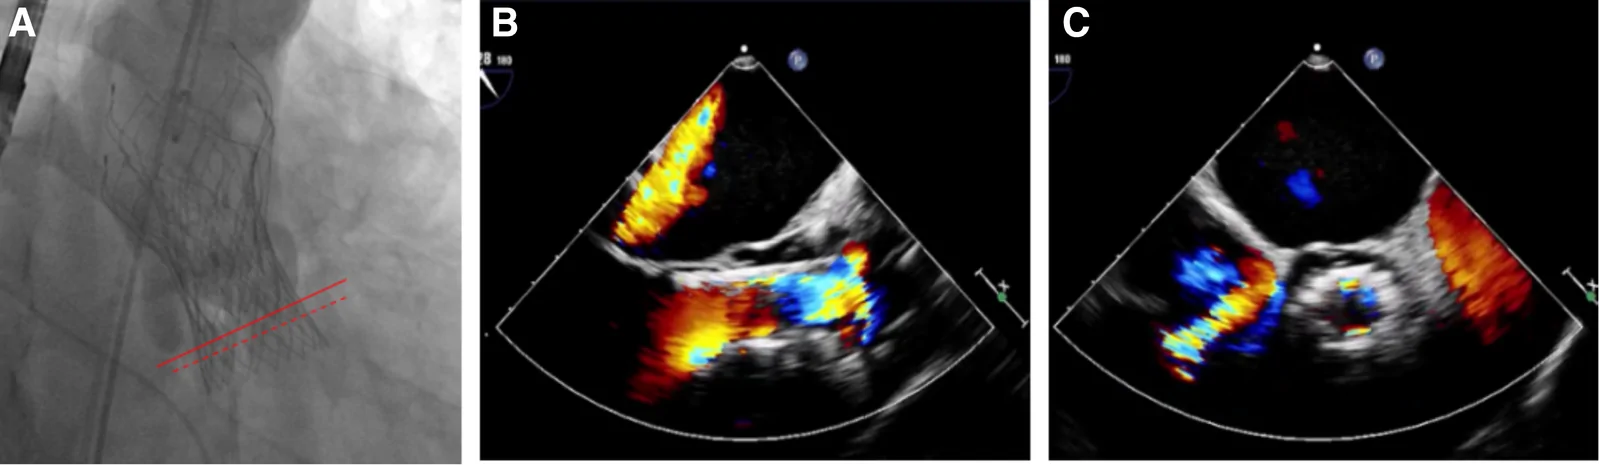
Postoperative digital subtraction angiography (DSA) and transesophageal echocardiography (TEE) evaluation. Digital subtraction angiography (DSA) and transesophageal echocardiography (TEE) evaluation. (A) The solid red line shows the plane of the valve ring, and the red dotted line shows the plane of the bottom of the 2nd valve prosthesis. (B and C) The TEE evaluation results after the 2nd TAVR operation showed that there was no PVL and central reflux in the long-axis plane and no reflux beam in the short-axis plane. PVL = paravalvular leakage, TAVR = transcatheter aortic valve replacement.
